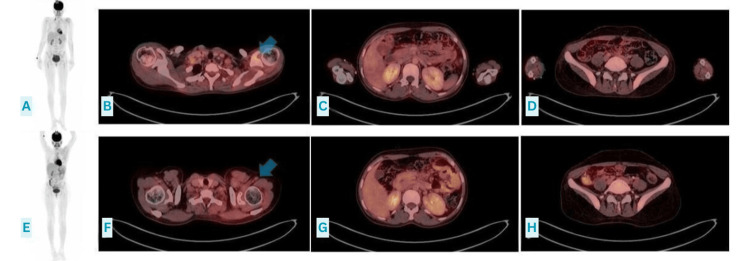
FDG PET/CT (A-D): Interim FDG PET/CT images obtained after four cycles of chemotherapy showing a very good partial metabolic response, with mild residual FDG uptake in the left shoulder region. (E-H): End-of-treatment FDG PET/CT images obtained after six cycles of chemotherapy and completion of consolidative radiotherapy to the left shoulder, demonstrating complete metabolic response with no evidence of residual hypermetabolic disease. FDG PET/CT: fluorodeoxyglucose positron emission tomography/computed tomography
